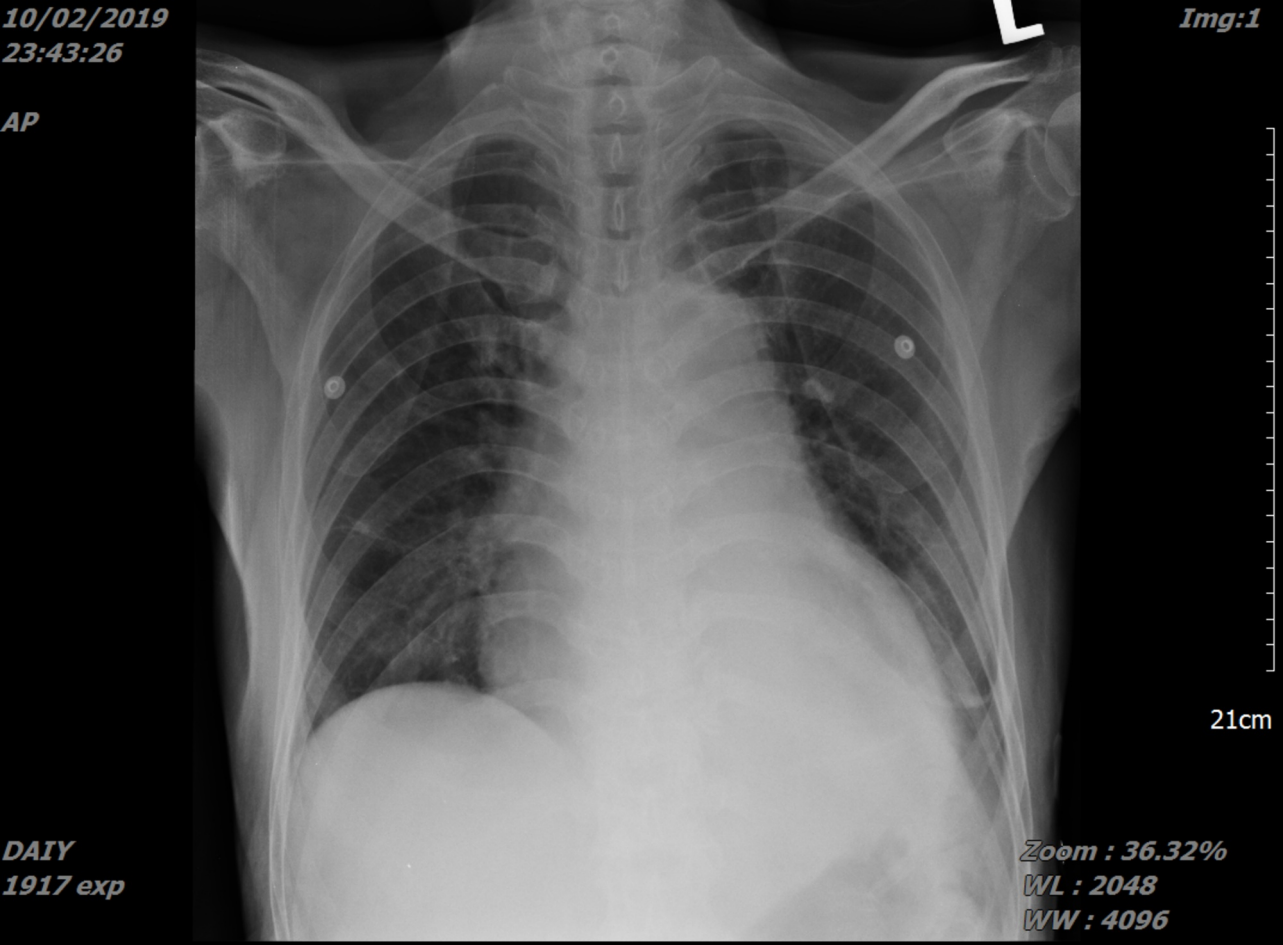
Chest radiograph after emergency pericardiocentesis.
